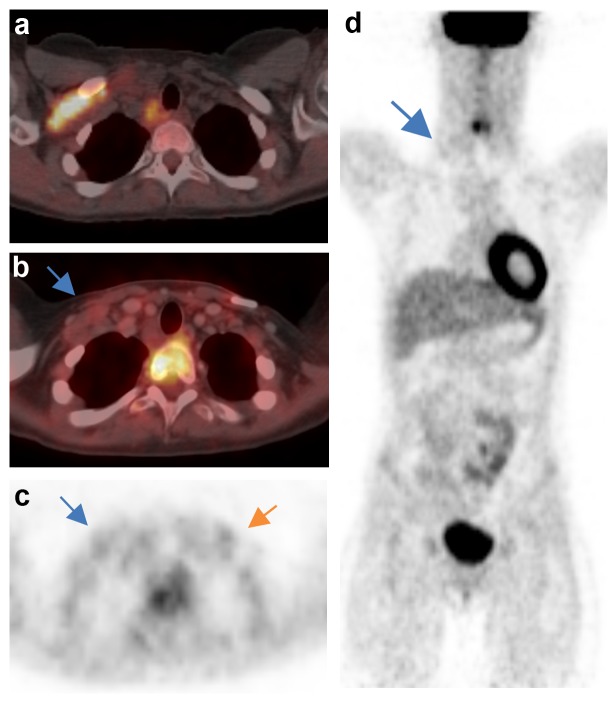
Discrepant D scoring of the right supraclavicular region. Case 63. a) Tumour involvement before treatment. b) Residual mass in this region after two cycles of OEPA. c) and d) slightly enhanced FDG uptake above background in this area. Interpretation might be complicated by a slightly enhanced FDG uptake symmetrically in the left supraclavicular area which, however, is in the surrounding of a catheter and by the inhomogeneous uptake in the mediastinum. Deauville score of the readers R1-5, respectively: 1-1-3-1-2. Courtesy Dept. Nuclear Medicine, Karolinska University Hospital, Stockholm, Sweden.
